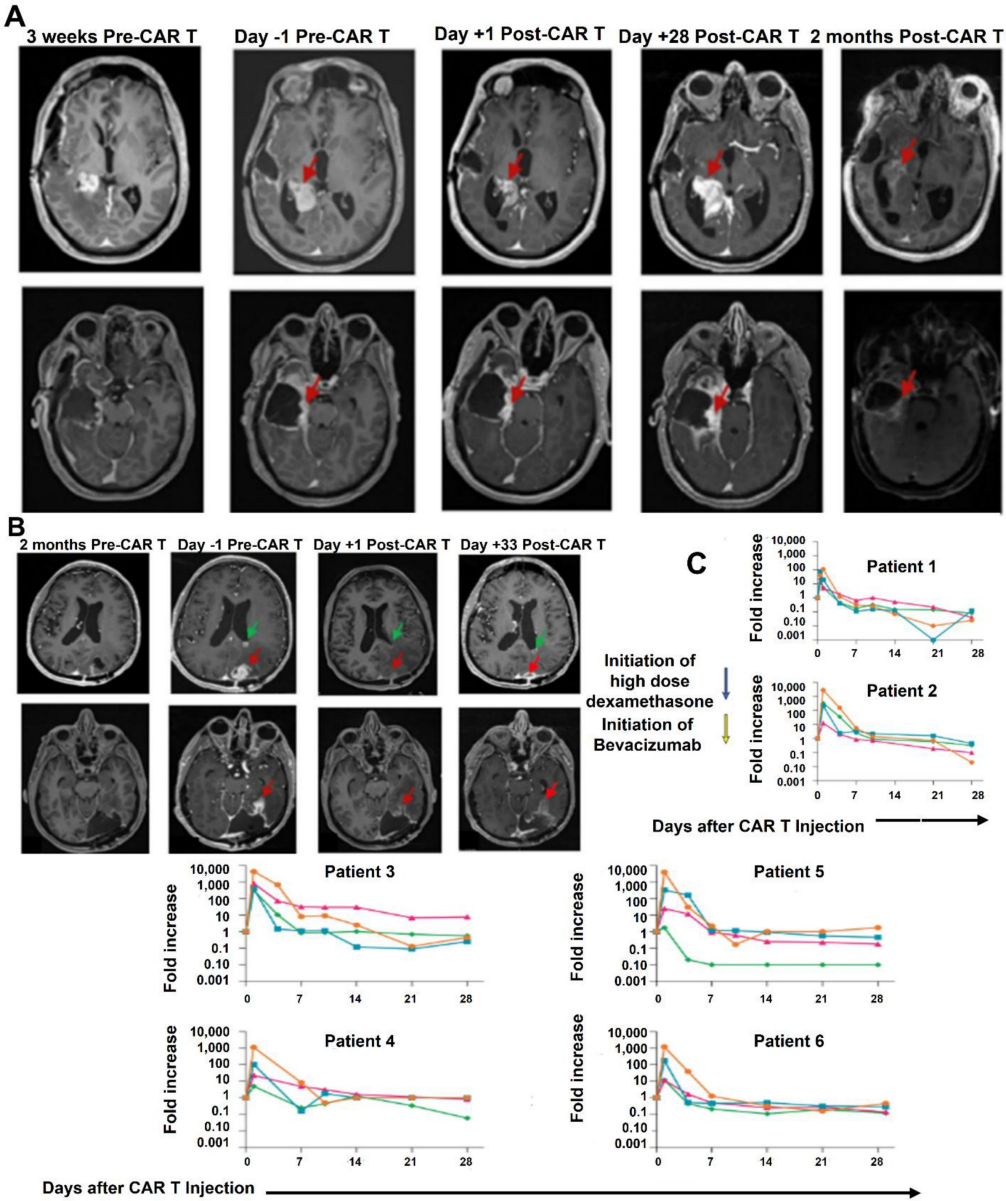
Multifocal rGBM regression upon intraventricular administration of CART-EGFR-IL13Rα2 cells (dose level 1). (A) Patient 2: A posterior mesial temporal lobe nodule (2.7 × 1.9 × 2.7 cm) grew significantly over three weeks. Post-CAR T therapy, nodule size and enhancement initially decreased but later expanded by day +28. Without further treatment, subsequent scans revealed regression of the nodule and stabilization of resection cavity enhancement. (B) Patient 1: Axial gadolinium-enhanced T1-weighted images revealed a solidly enhancing left parieto-occipital nodule (red arrow) and a necrotic nodule near the left lateral ventricle ependyma (green arrow) that developed over two months. Post-CAR T cell infusion, the prominent lesion receded within 24 hours (red arrow). By day +33, improvement continued with central necrosis at the lesion site, though spatial enlargement of the left occipital resection cavity (red arrow) was noted and surgically removed. Treatment-related alterations were seen in 90% of tissue samples. (C) Cytokine data (IFNγ, IL-2, TNFα, IL-6) showed peaks within 1-4 days post-CAR T infusion, reverting to baseline by day 14. High-dose dexamethasone was initiated 10-24 hours post-injection Adapted with permission from reference 104 Copyrights 2024, SPRINGER NATURE.
